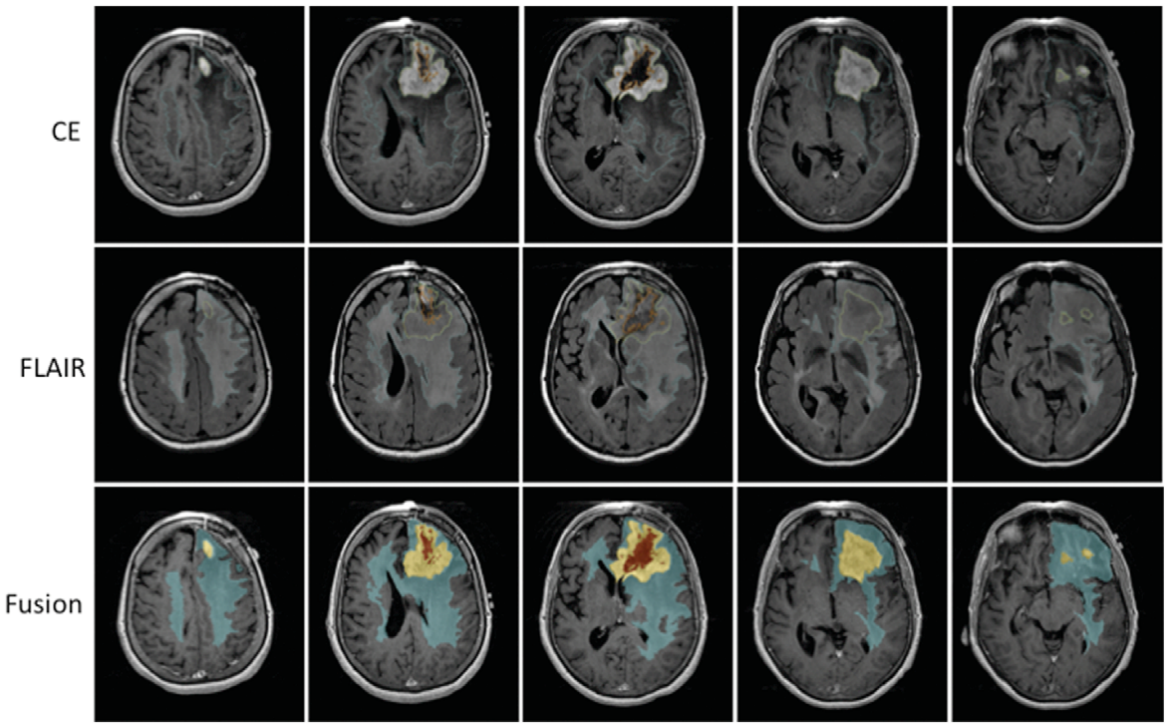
High FLAIR patient. A 59 year old female patient with a left frontal GBM. The segmented edema/tumor infiltration (blue), enhancement (yellow) and necrosis (orange) are seen overlaid on a base post- contrast T1WI. The images demonstrate a patient with a medium to large GBM with a relatively small amount of peritumoral FLAIR hyperintensity reflecting edema and tumor infiltration.
